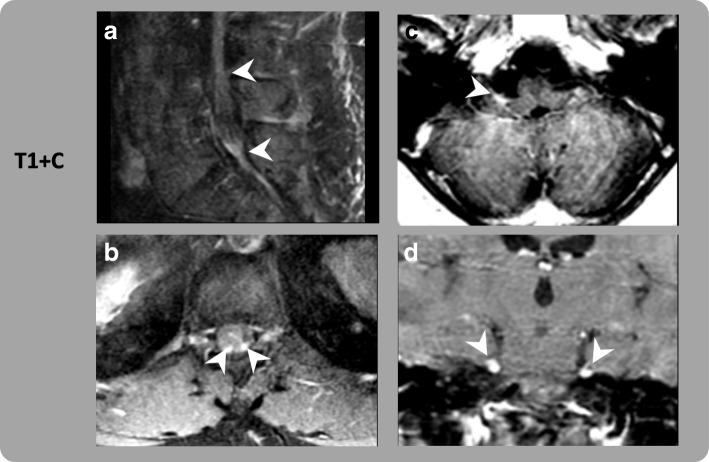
Gadolinium Enhanced MRI Brain and Spine, Images of Patient 2. Post-contrast MR images demonstrate abnormal leptomeningeal enhancement throughout the CNS. (a) abnormal smooth thickening and enhancement of the cauda equina and nerve roots (arrowhead). (b) abnormal smooth thickening of the nerve roots at the thoracolumbar junction (arrowhead). (c) abnormal enhancement of the 7^th^ cranial nerve (arrowhead). (d) abnormal enhancement of both 5^th^ cranial nerves seen in a coronal plane (arrowhead)
